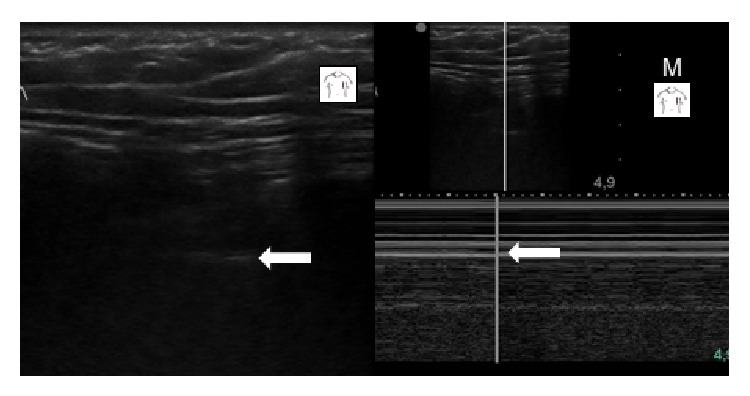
Case 1: B-mode and M-mode sonography using a linear transducer positioned in the mid-clavicular line at the 3rd left intercostal space. Arrows indicate the pleural lines (B: B-mode, M: M-mode).
